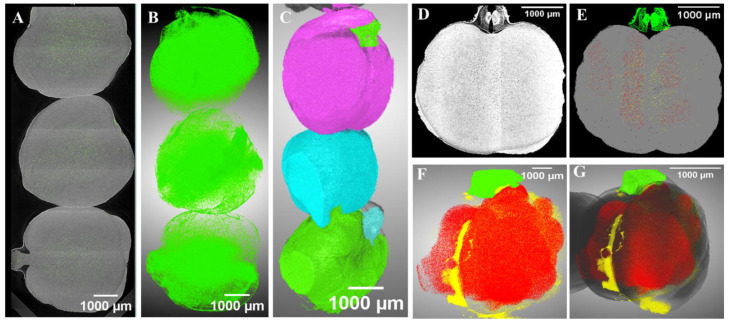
(A): Reconstructed vertically stitched X-ray image of three control chickpea seeds with labeled pores (green), (B): labeled pores in 3D visualization, (C): each labeled seed represents its volume, (D): X-ray reconstructed image of chickpea (K9mc-16wk-30t), (E): labeled (germ: green, change due to deterioration: red, pores: yellow and endosperm: grey), (F): 3D visualization of labeled microstructural features, and (G): full seed 3D view with all labeled features. (*n* = 1, single kernel was used in image processing).
